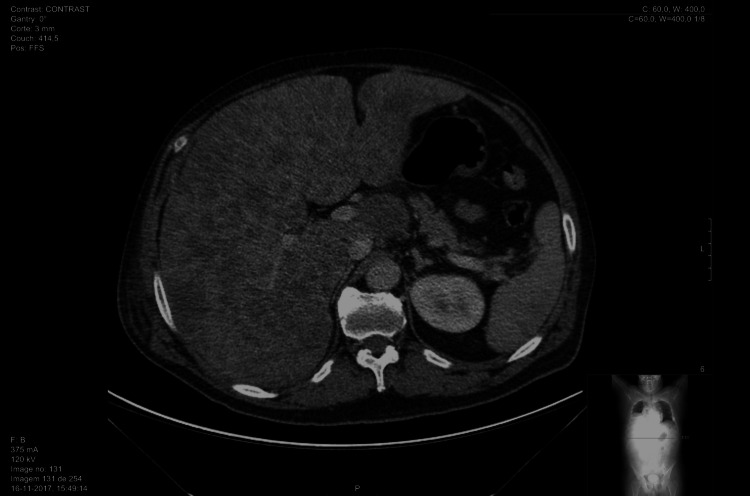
Full-body CT scan showing hepatic lesions.
